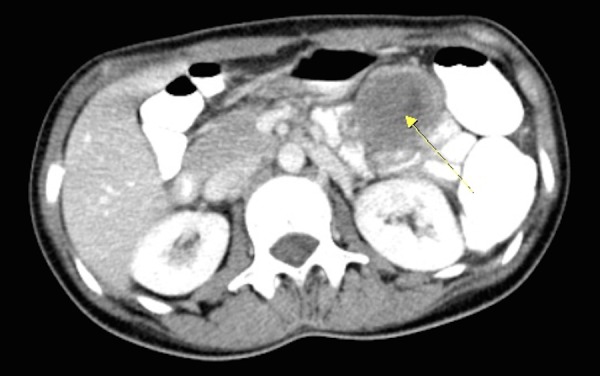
The Lesion (arrow) Appearing on Computed Tomography (CT)
